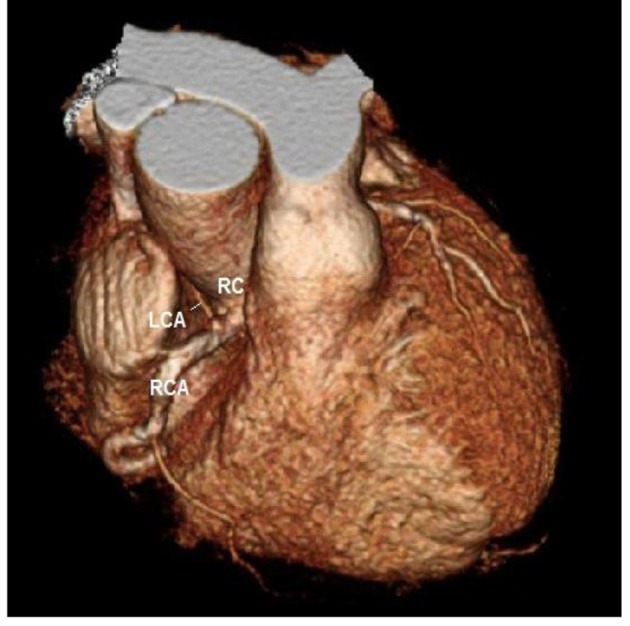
Coronary CT angiogram showing the RCA and LCA originating from the right coronary cusp.
